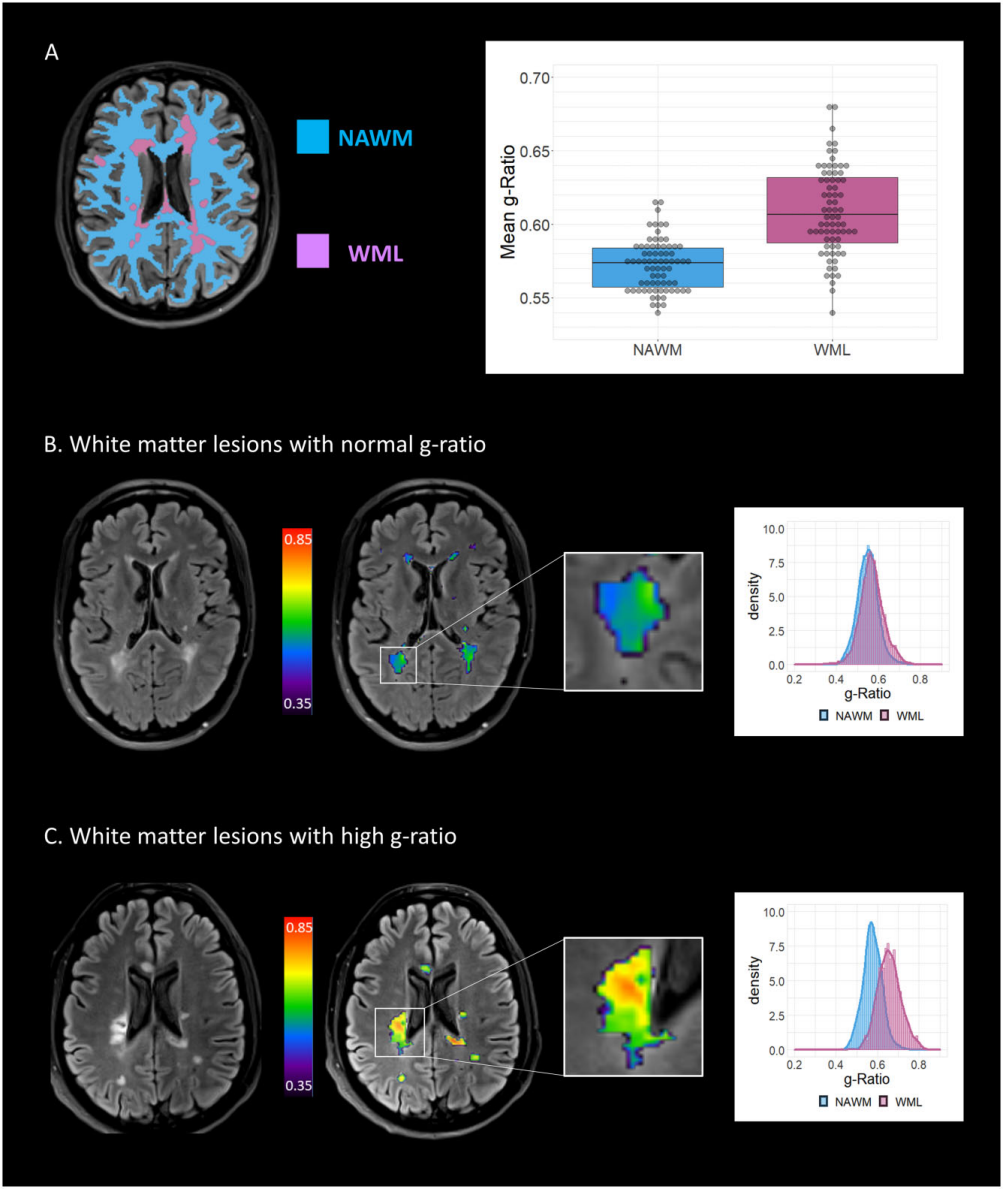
G-ratio in cerebral white matter lesions at MS diagnosis. A shows segmentation strategy for anatomical MRI scans, dividing white matter into white matter lesions (WML, purple) and normal-appearing white matter (NAWM, blue). The g-ratio is increased in lesions compared to NAWM in relapsing–remitting multiple sclerosis patients (0.61 versus 0.57, difference: 0.036, 95% CI: 0.029–0.043, *P*<0.001 paired t-test). B shows representative patient with normal g-ratio within WML. On the left, the T_2_ Fluid-Attenuated Inversion Recovery image is shown, with hyperintense WML consistent with multiple sclerosis. The aggregate g-ratio from these lesion segmentations was evaluated (right-hand MRI, g-ratio denoted by colour scale), leading to a histogram where the distribution of g-ratio values between NAWM (blue) and WML (purple) could not be distinguished. C shows representative patient with high g-ratio within WML. The lesion shown has a high g-ratio (right-hand MRI) and the resulting individual histogram from the patient shows separation of g-ratio distributions derived from NAWM (blue) and WML (purple).
